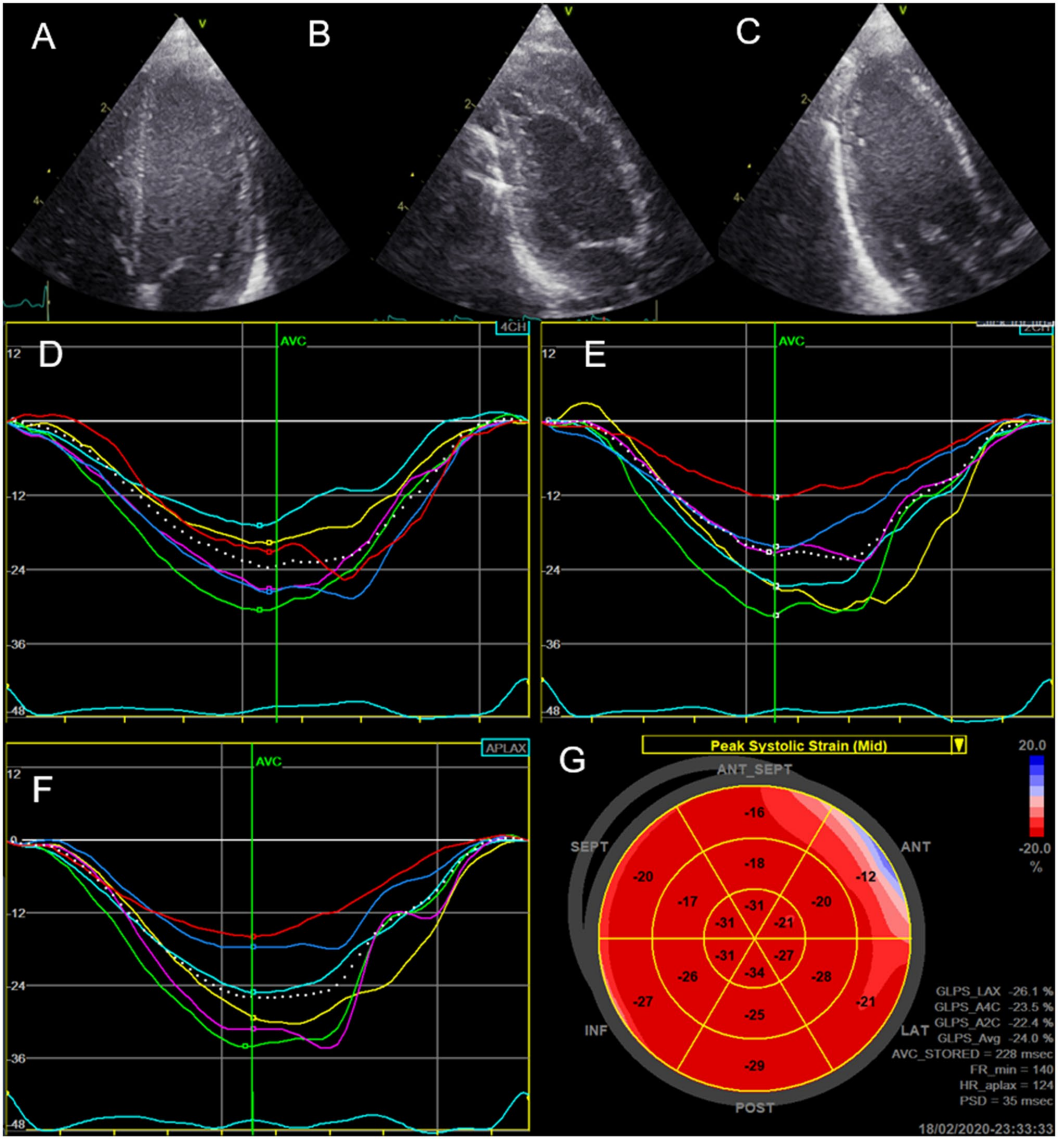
Speckle tracking echocardiography derived strain measurements from all three views, their respective strain curves and a combined bullseye plot showing segmental strain values in the midwall (corresponding graphs were also obtained for the endocardial and epicardial layers). A Four chamber view; B two chamber view; C three chamber view; D strain curves four chamber view; E Strain curves two chamber view; F strain curves three chamber view; G Bullseye plot presenting segmental strain values
